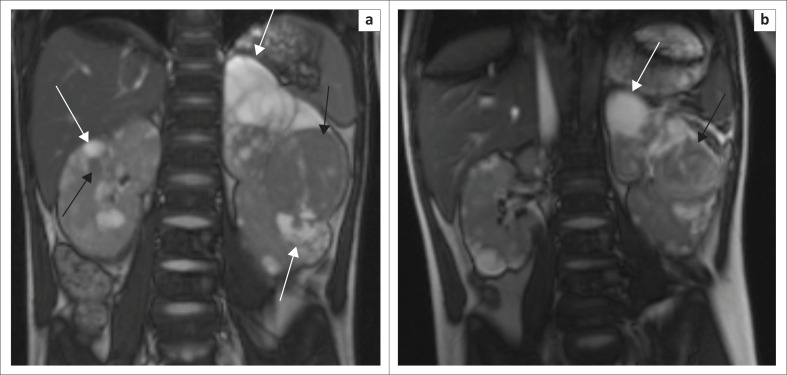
(a) Prior to treatment. T2-weighted coronal. Enlarged left kidney with multiple bilateral hyperintense (white arrows) and more hypointense masses (black arrows). (b) T2-weighted TRUFI coronal post-sirolimus and radiofrequency ablation of the left midpole renal cell carcinoma, with size reduction of left upper pole (white arrow) and midpole renal cell carcinoma ( black arrow).
